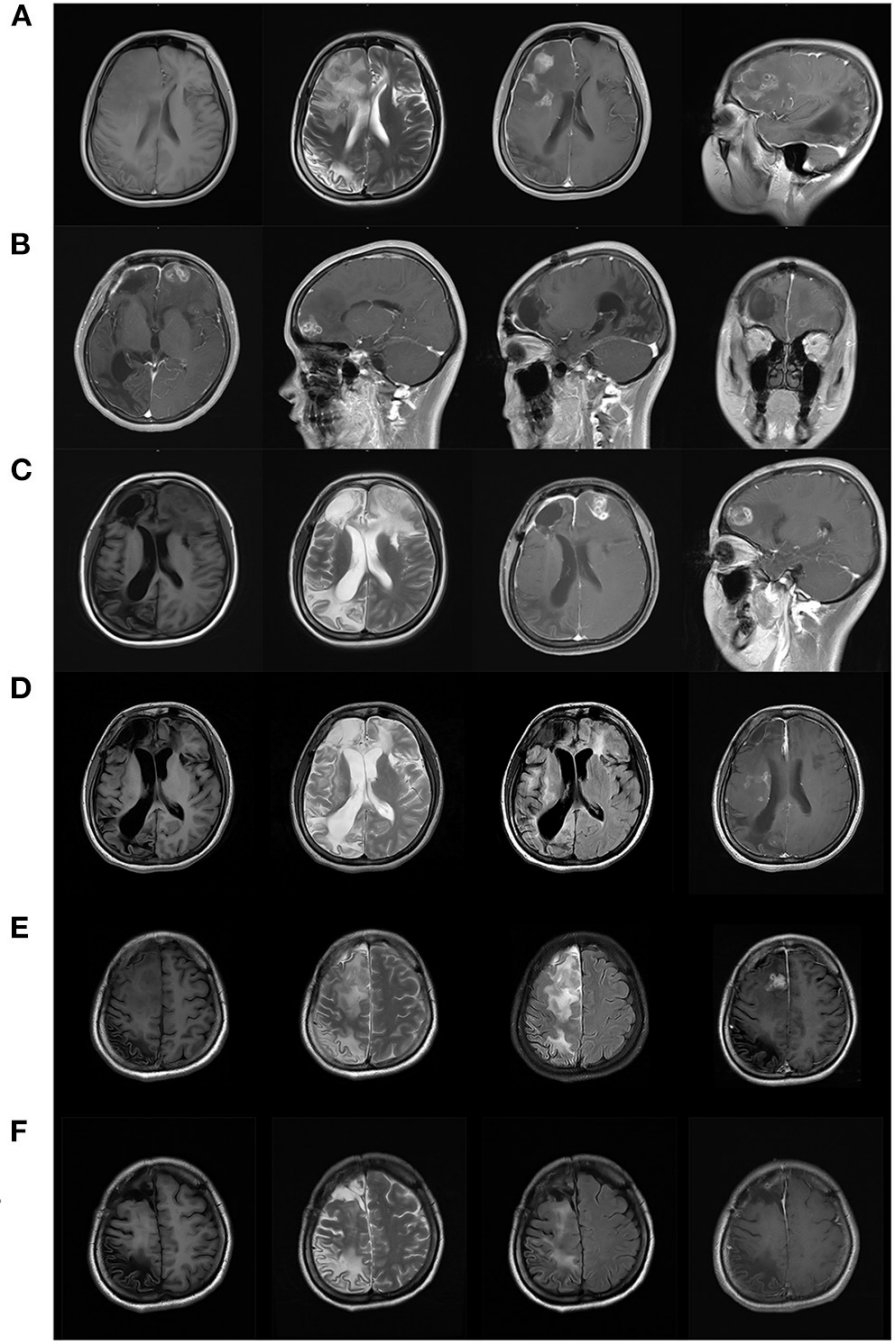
Neuroimaging results of cerebral sparganosis in the case. (A) Six years after the onset (aged 22), tumor-like occupation foci at the right frontal lobe with edema and irregular enhancement causing midline shift on MRI before the first operation. Note that regular ring-shaped enhancement appeared on the sagittal view (the rightmost figure) (Left to right: T1, T2, axial T1 post gad, sagittal T1 post gad). (B) Three months after the operation, the enhanced foci shifted to the left frontal lobe, and postoperative encephalomalacia occurred at the original right frontal lobe on her follow-up MRI images (Left to right: axial T1 post gad, sagittal T1 post gad (left side), sagittal T1 post gad (right side), coronal T1 post gad). (C) Five months after the operation, a typical tunnel sign at the left frontal lobe was found on both axial and sagital view of her repeated MRI with enhancement (Left to right: T1, T2, axial T1 post gad, sagittal T1 post gad). (D) Three years after the operation (aged 25), the patient came to our center with left side paralysis. Restricted enhancement foci in the right centrum semiovale and basal ganglia with punched-out and tunnel-like presentation were found at the right basal ganglia (Left to right: axial T1, T2, T2 FLAIR, T1 post gad). (E) Four years after the operation (aged 26), the larva moved to the superficial area of the right frontal lobe where surgical removal became possible (Left to right: axial T1, T2, T2 FLAIR, T1 post gad). (F) 16 months after the removal operation (aged 28), MRI revealed softened foci, gliosis and shrinkage of the parenchyma at the right frontal and parietal lobe. No focal or meningeal enhancement was found (Left to right: axial T1, T2, T2 FLAIR, T1 post gad).
